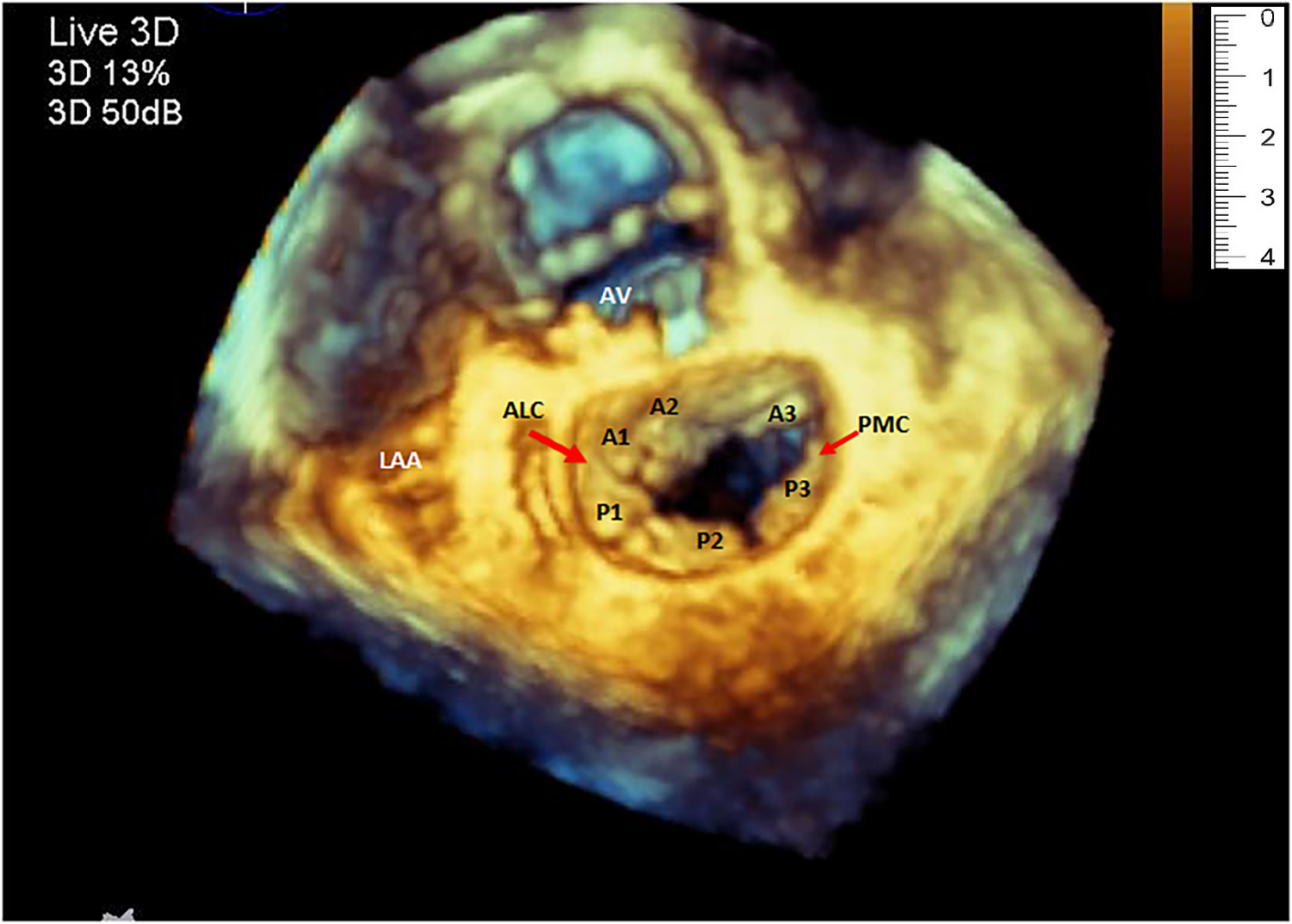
Three-dimensional echocardiography image of the mitral valve showing the normal shape of the annulus, both leaflets and the corresponding segments. (LAA, left atrial appendage; ALC, antero-lateral commissure; PMC, postero-medial commissure).
